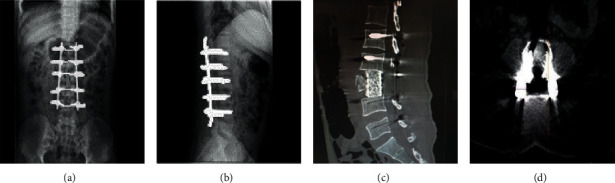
GSICT  scan images processed by GSI and MARS. (a) Coronal position of lumbar vertebra. (b) Sagittal position of lumbar vertebra. (c) Sagittal position of thoracic vertebra. (d) Transverse position of lumbar vertebra. Compared with conventional CT  scan images, the image clarity of the above four figures was obviously improved, and metal radial artifacts were basically eliminated. The relationship among artificial joints and the position of internal fixation nails were shown to facilitate observation.
